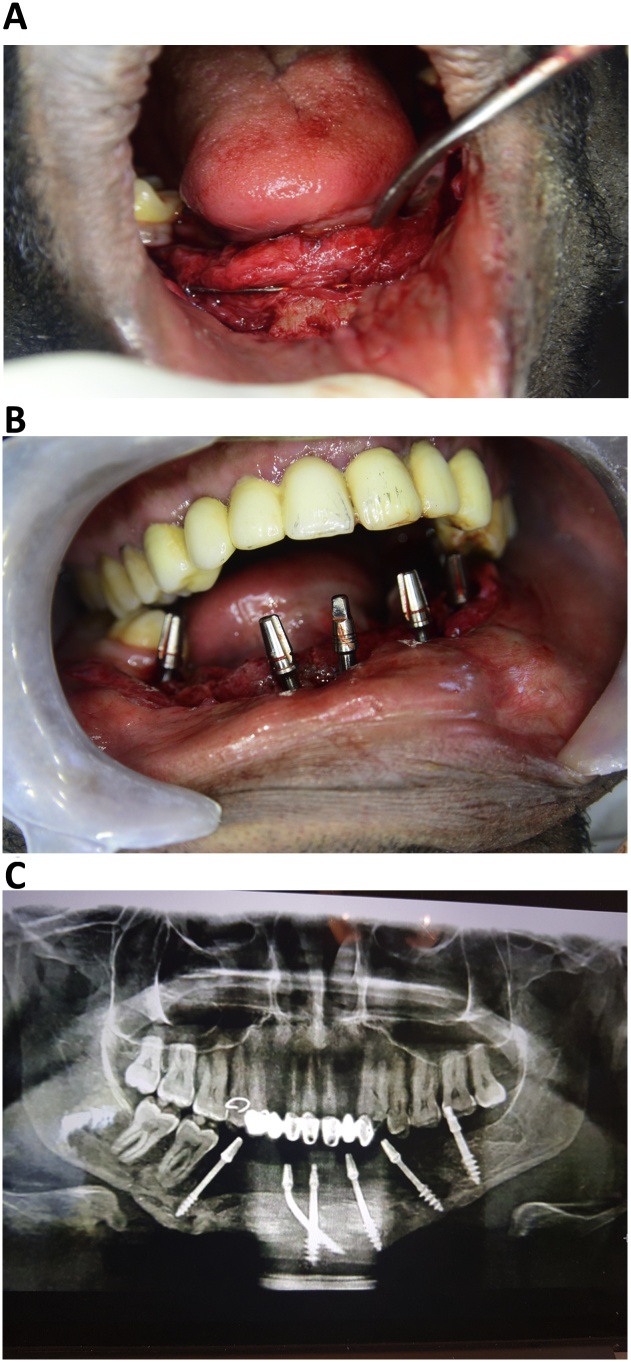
Intraoral rehabilitation of a 32-year-old man with a gunshot defect using immediately loaded basal implant-supported fixed prostheses. A. Elevation of a periosteal flap, removal of the supporting wire, and implant insertion. B. Implant distribution and flap suturing. C. Post-operative panoramic radiograph.
